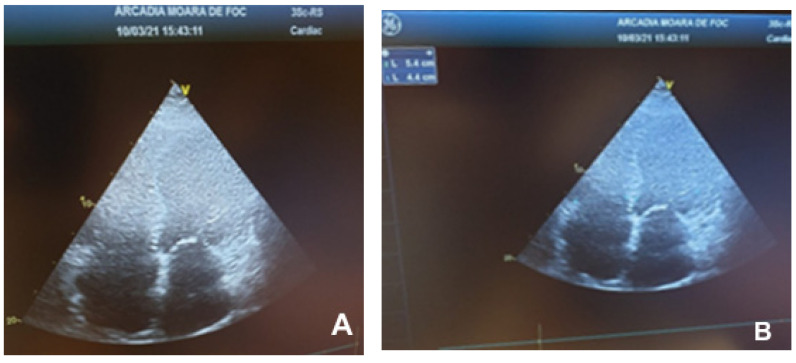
Dilated cardiomyopathy echocardiogram. (A,B)—Apical 4 chamber view with global cardiomegaly and abnormal sphericity index.
